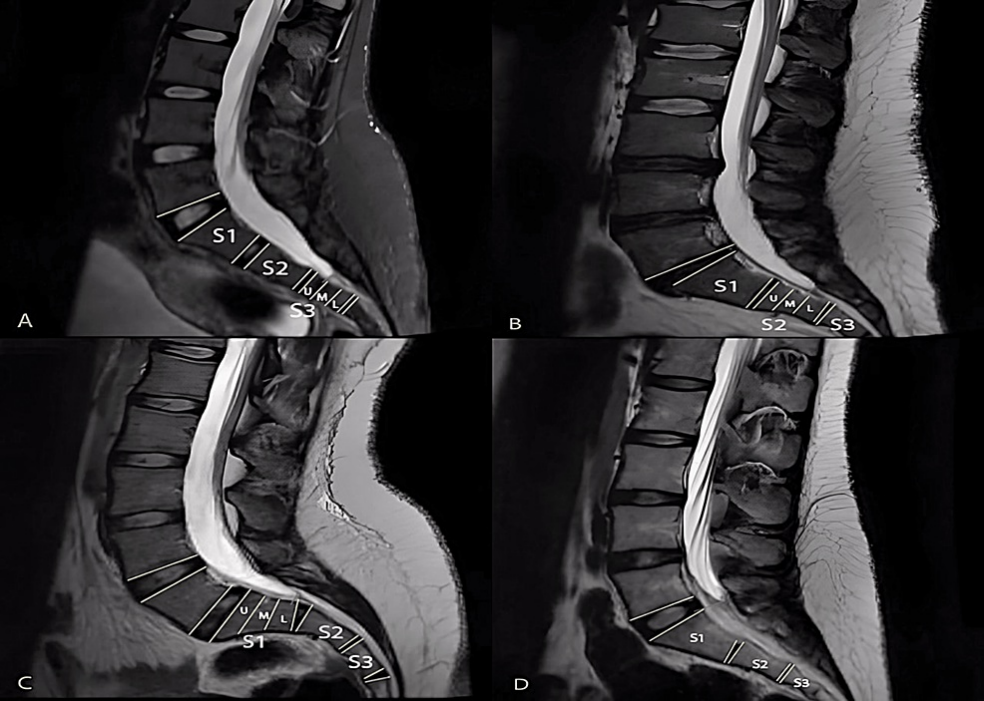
T2 weighted MRI images with various DS termination levels (A) The DS Tip at the S3 Upper level. (B) The DS Tip at the S2-Middle level. (C) The DS Tip at the S1-Lower level. (D) The DS Tip is at the space L5-S1 Disk level MRI: Magnetic resonance imaging; DS: Dural sac
